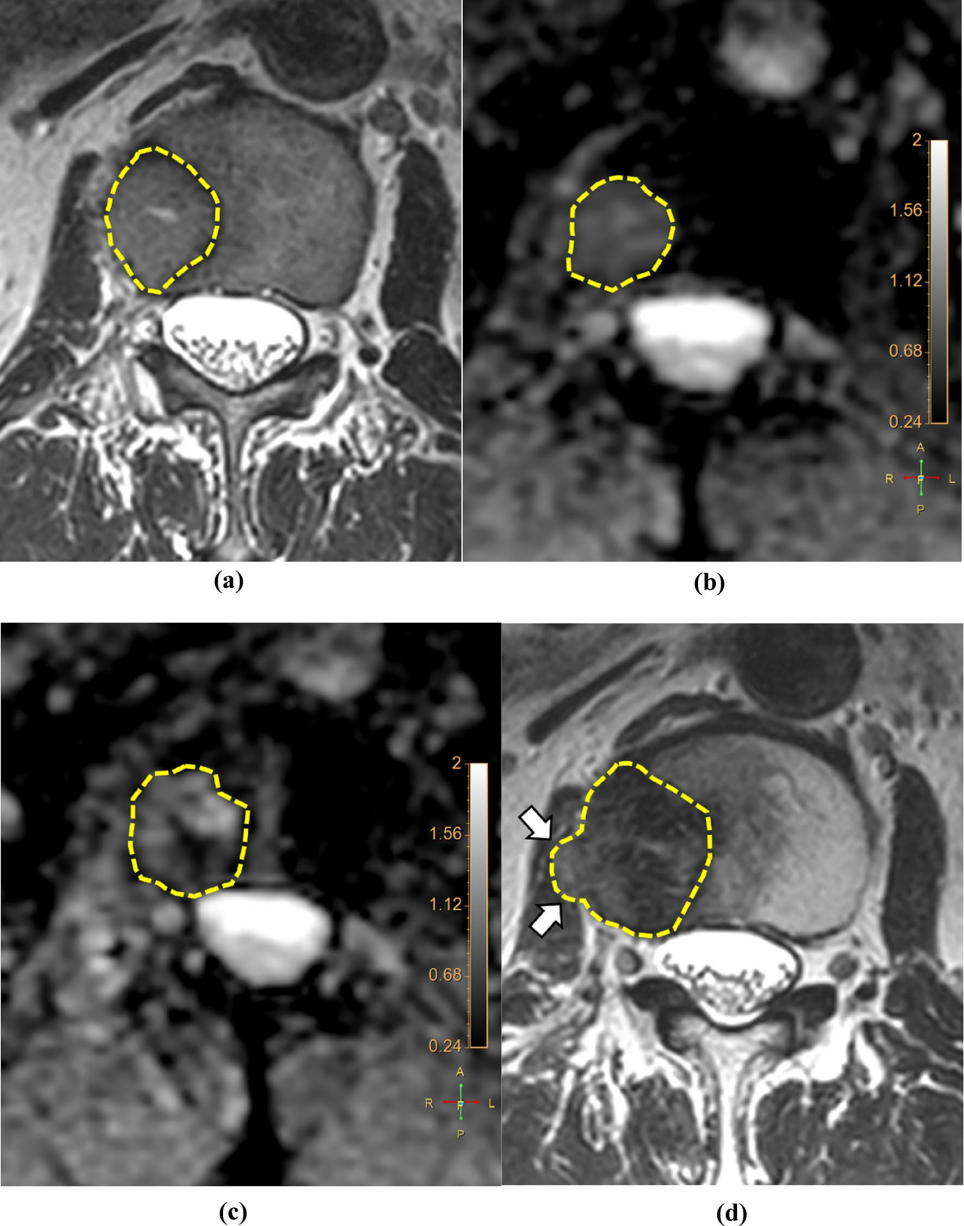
Images of a 72-year-old man with metastasis to the L2 vertebra from a hepatocellular carcinoma. The tumor margin is delineated by yellow dotted lines. (a) The T2WI of the baseline MRI shows a 1.8 × 2.3-cm-sized metastatic lesion. The average mean ADC values measured by readers I and II in (b) the baseline MRI and (c) the first post-RT MRI were 0.72 and 0.71 (× 10^–3^ mm^2^/s), respectively. (d) The T2WI of the second post-RT MRI shows an increase in the size of the lesion to 2.6 × 2.7 cm with extraosseous extension (arrows), representing PD. *ADC* apparent diffusion coefficient, *MRI* magnetic resonance imaging, *RT* radiation therapy, *T2WI* T2-weighted image, *PD* progressive disease.
